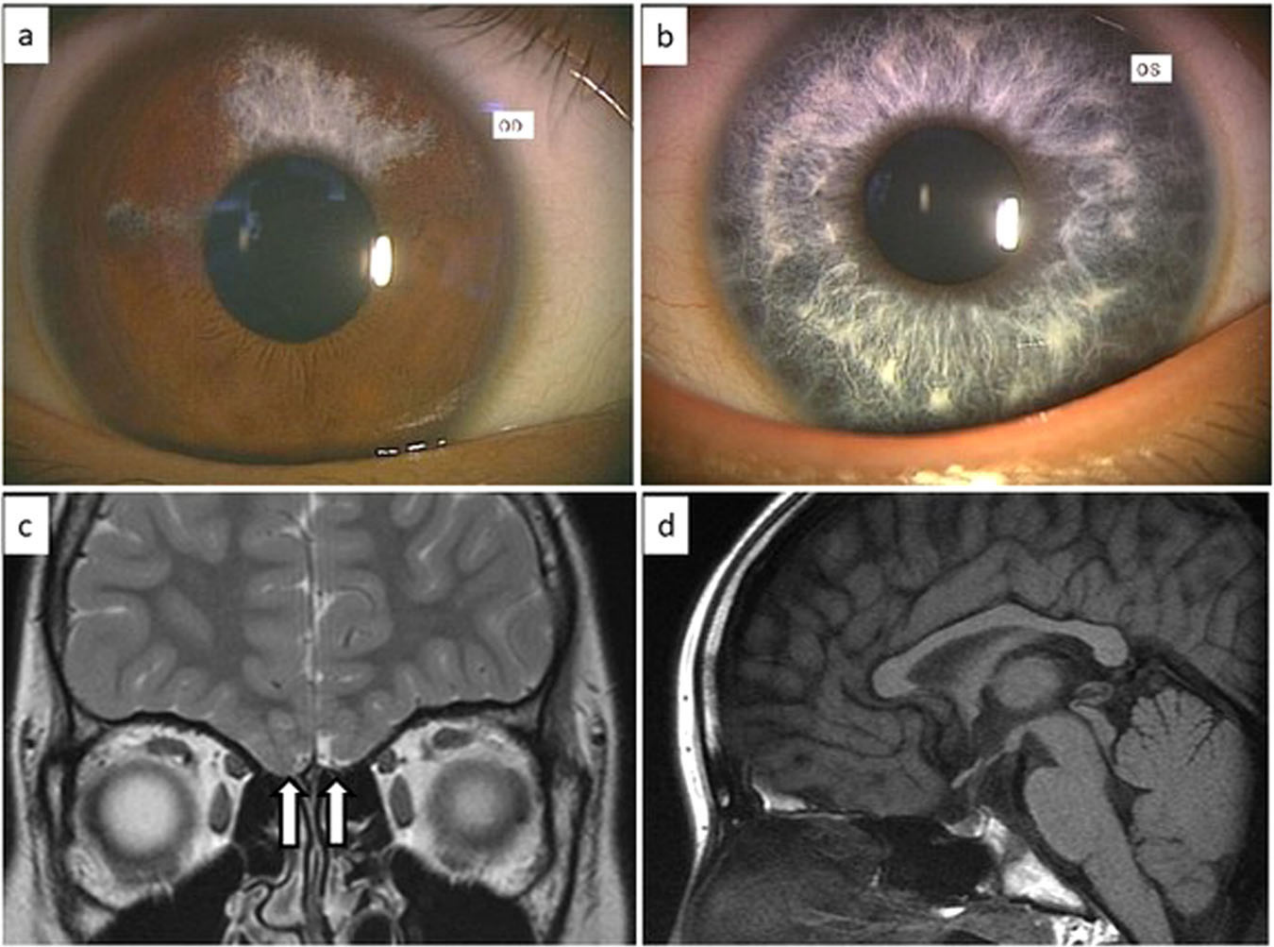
The picture of iris, and MRI findings. a, b Iris depigmentation. a Right (partial depigmentation). b Left (complete depigmentation). c, d T2-weighted brain magnetic resonance imaging. c White arrows show olfactory bulb agenesis. d No abnormalities were noted in the hypothalamus and pituitary.
